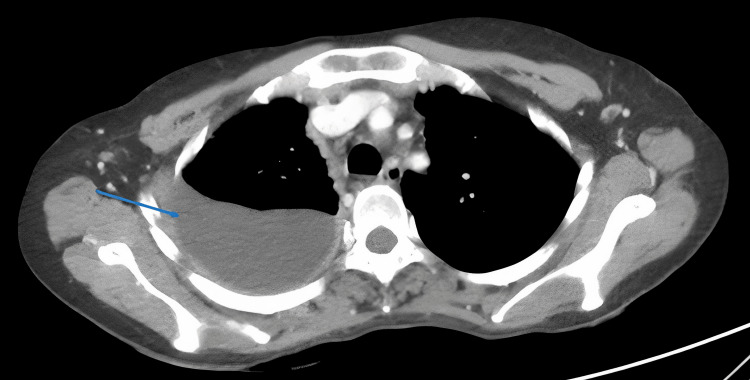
Contrast-enhanced CT scan of the chest showing right-sided pleural effusion (blue arrow).
